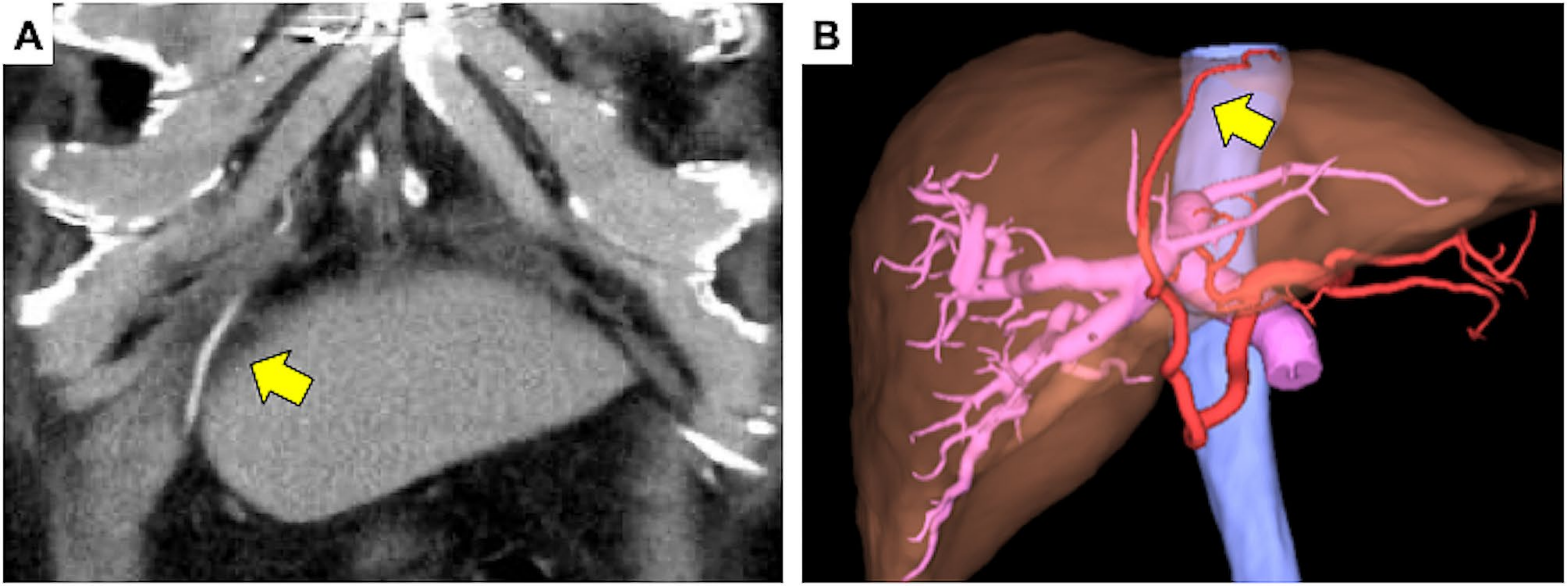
Enhanced abdominal computed tomography (CT) and SYNAPSE VINCENT results. Enhanced CT (A) and SYNAPSE VINCENT (B) shows the right gastroepiploic artery graft along the hepatic falciform ligament (arrow). CT: computed tomography
